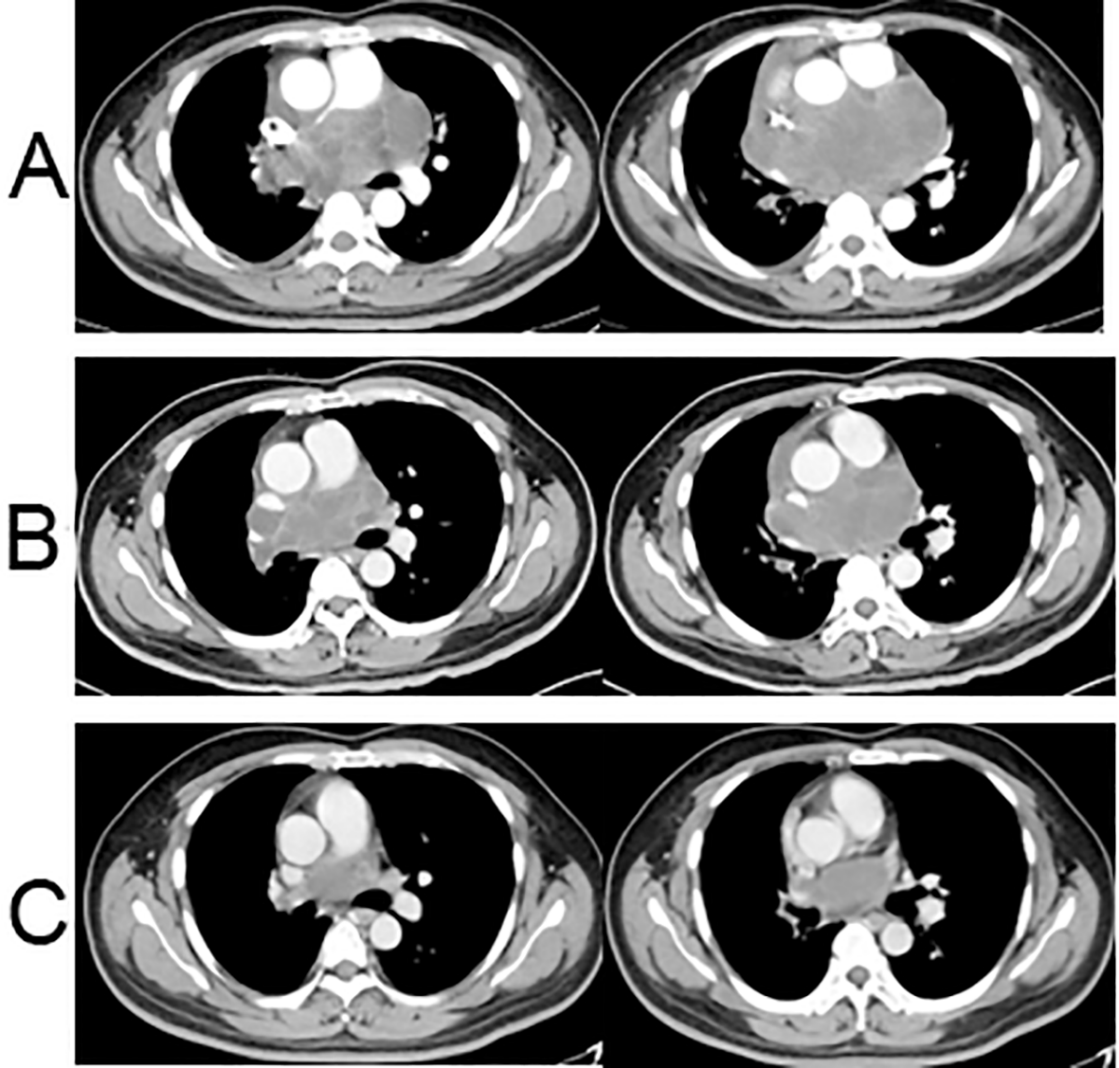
Chest CT scans after neoadjuvant treatment. (A) Chest CT enhanced scan after one cycle of chemotherapy: the tumor had increased in size, measuring 14.0 × 7.5 cm; (B) Chest CT enhanced scan after two cycles of chemotherapy + anlotinib: the tumor had significantly shrunk with a size of approximately 10.1 × 6.3 cm; (C) Enhanced chest CT scan after five cycles of chemotherapy + anlotinib: the tumor had significantly shrunk at the end of the fifth cycle of chemotherapy + anlotinib, measuring 6.8 × 3.7 cm.
